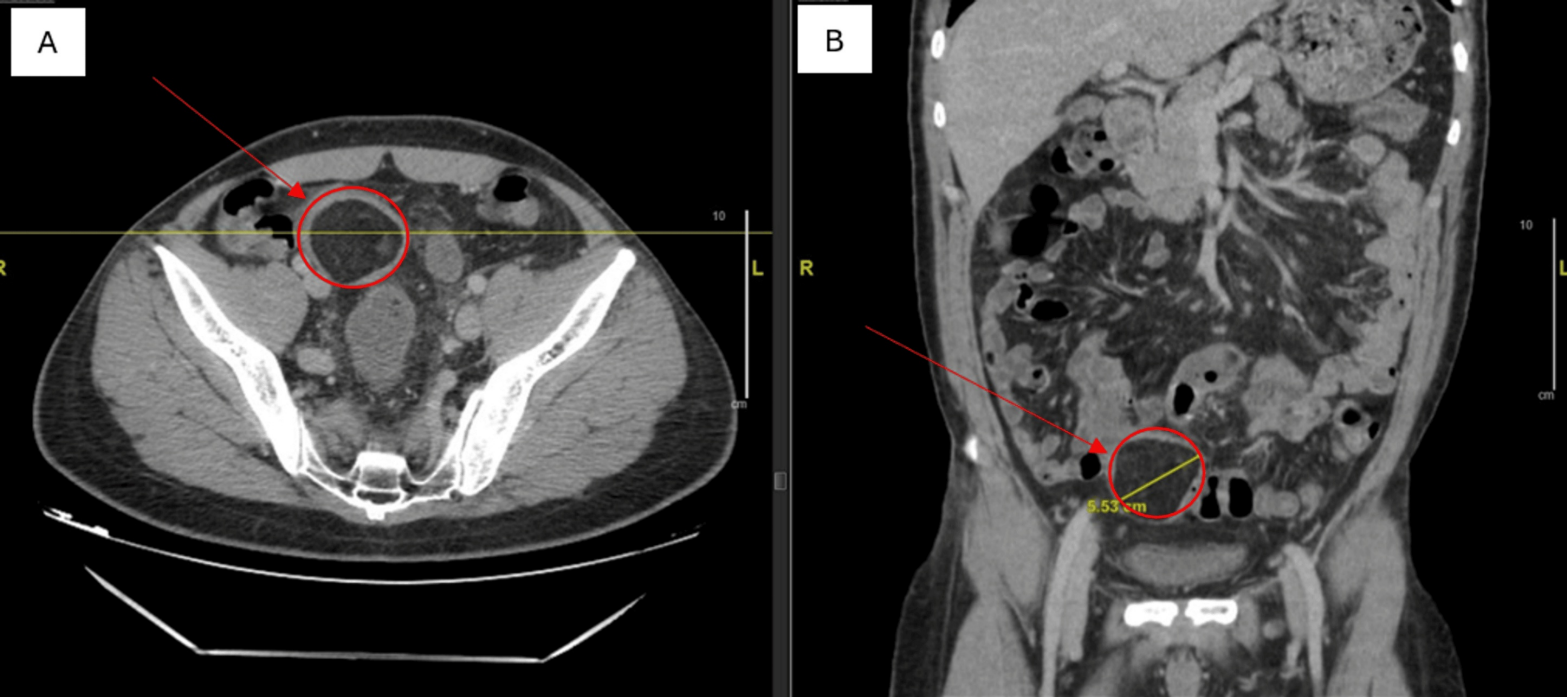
CT scan revealed an intramural lipoma in the sigmoid colon that measured 5.5×4.7×4.6 cm Panel A is an axial slice of the CT scan. Panel B is a coronal slice of the CT scan. In both panels A and B, the lipoma is indicated within the red circle and arrow.
